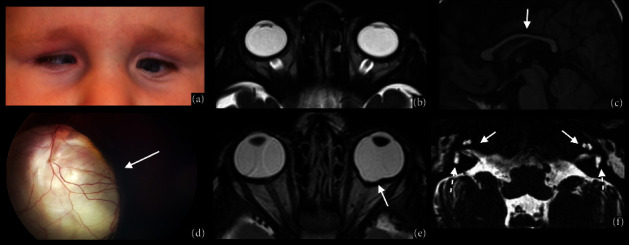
Simplex microphthalmia and complex colobomatous microphthalmia. Bilateral congenital microphthalmia in a one-year-old boy affected by 16q22 deletion syndrome (a–c). MRI T2WI shows bilateral primary microphthalmos, characterized by reduced anteroposterior and transverse ocular globe diameters (b). Sagittal T1WI of the brain shows associated corpus callosum hypoplasia (white arrow in (c)). RET-cam image of the left eye shows microphthalmia with a large chorioretinal coloboma involving the optic nerve (white arrow in (d)) in a two-year-old girl with CHARGE syndrome. Axial T2WI shows left ocular microphthalmia with typical posterior coloboma (white arrow in (e)). Brain MR with a dedicated DRIVE sequence demonstrates bilateral cochleovestibular dysplasia typically observed in CHARGE syndrome (white arrows in (f)).
